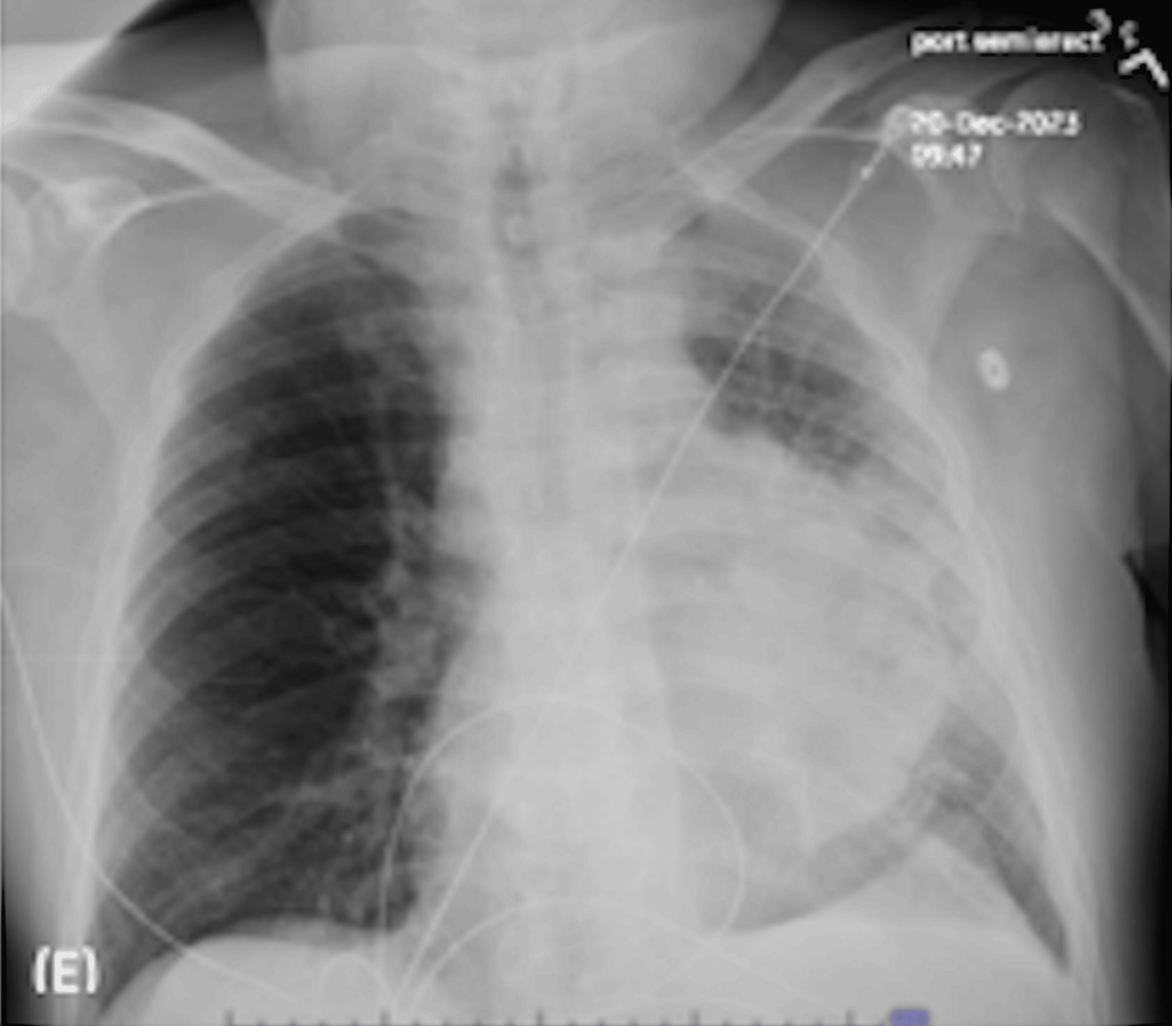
Post-procedure chest X-ray.
